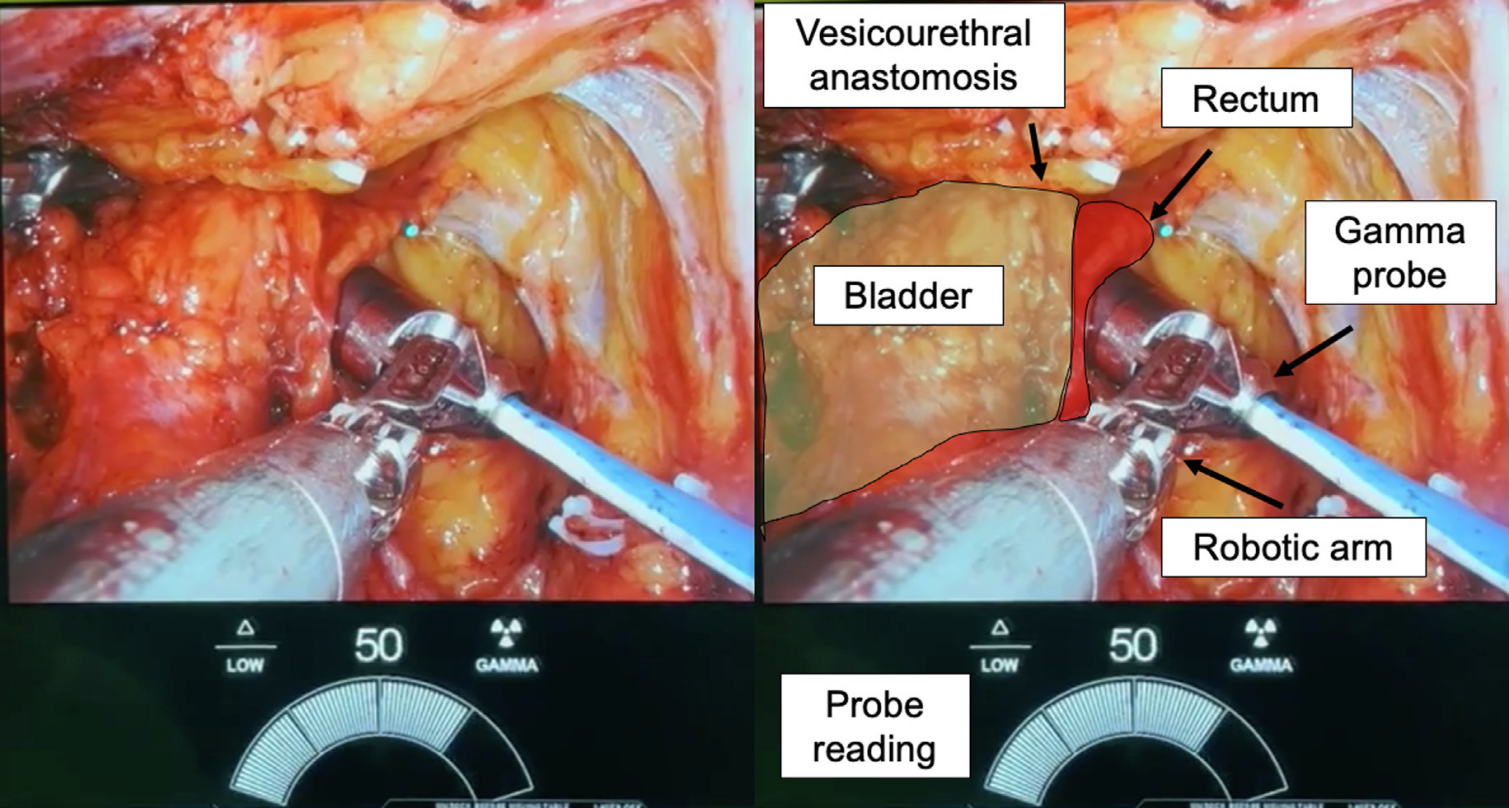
Intraoperative image of surgeon using a drop-in gamma probe. Image of surgeon’s view on robot console without (left) and with (right) labels. Probe reading was suggestive of a positive target in a patient with a known right perirectal target on PSMA PET. PET = positron emission tomography; PSMA = prostate-specific membrane antigen.
